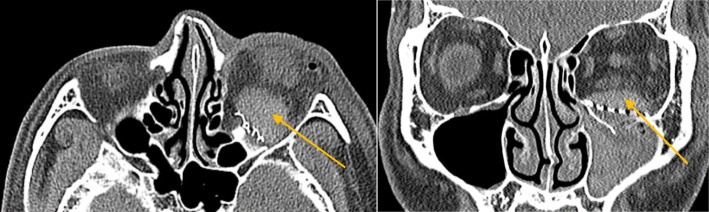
Postoperative CT (axial and coronal sections) showing retrobulbar hematoma (yellow arrows)
